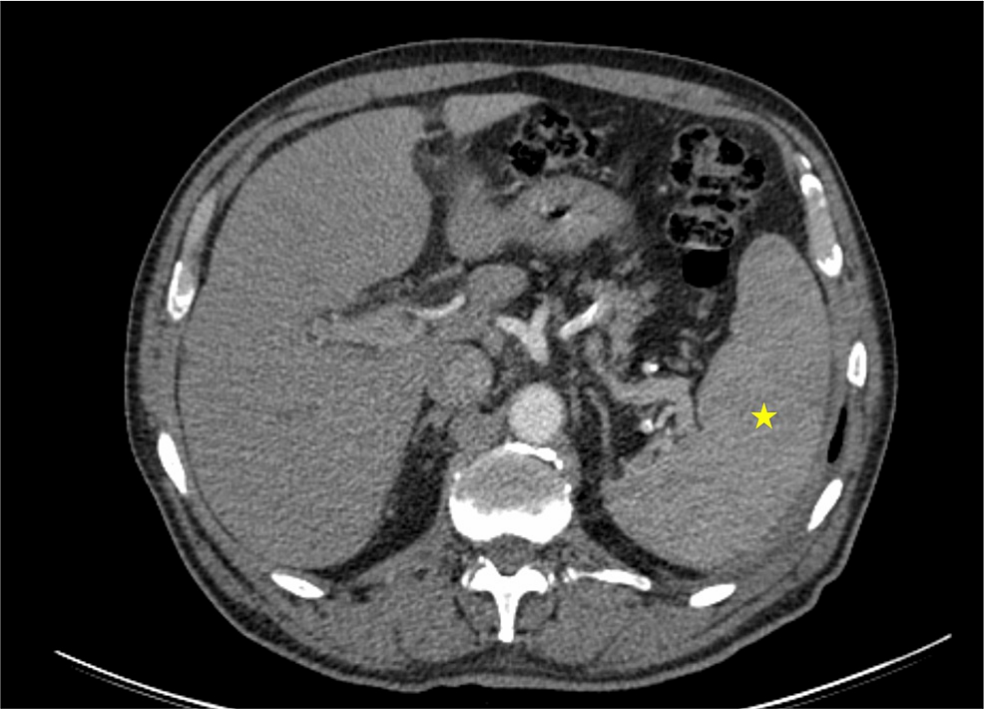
Abdominal CT Abdominal CT scan showing a 15 cm splenomegaly (yellow star), with no other significant findings
